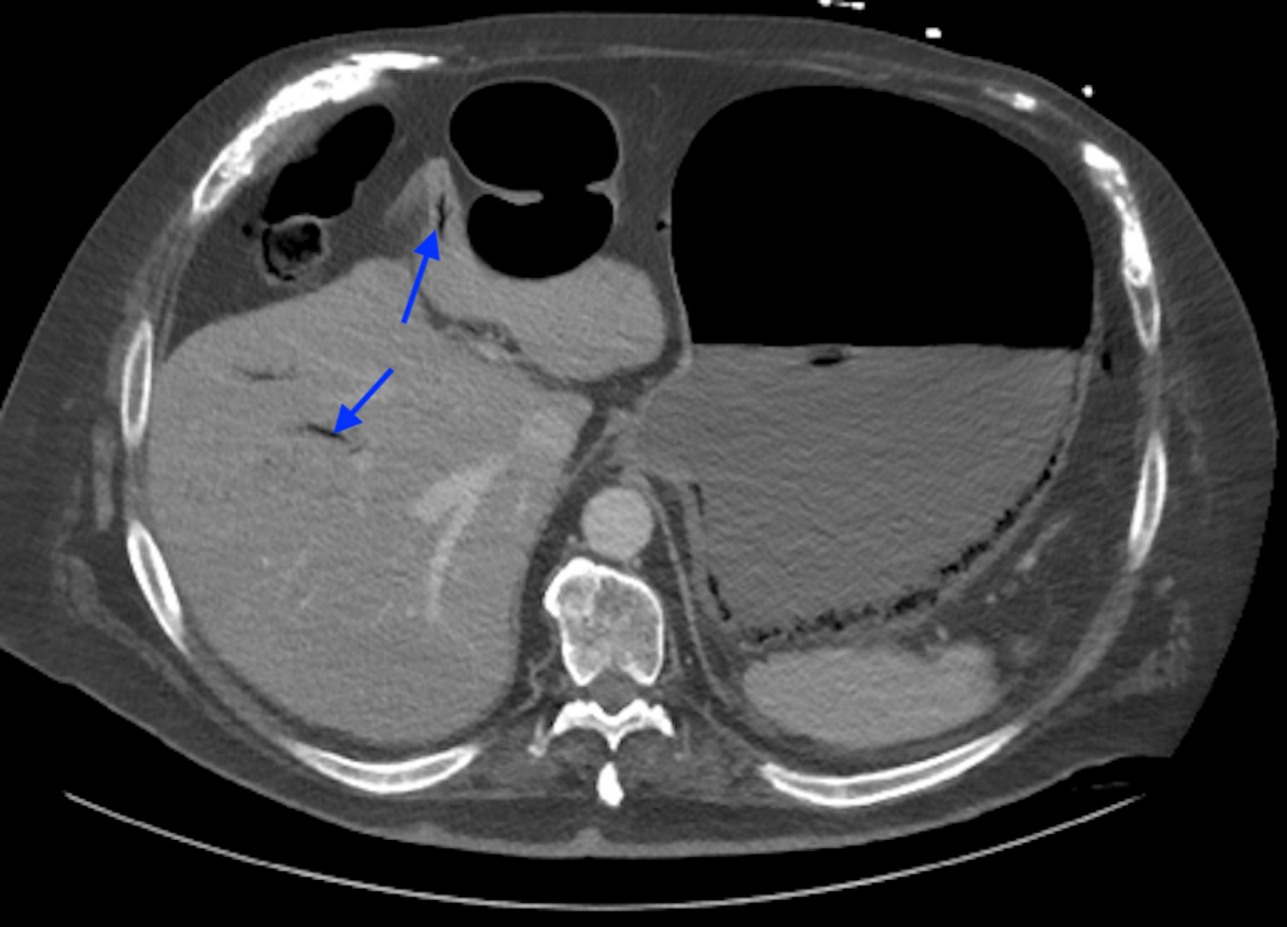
Axial CT of the abdomen demonstrating gas in the portal and perigastric veins (arrows). CT, computed tomography
